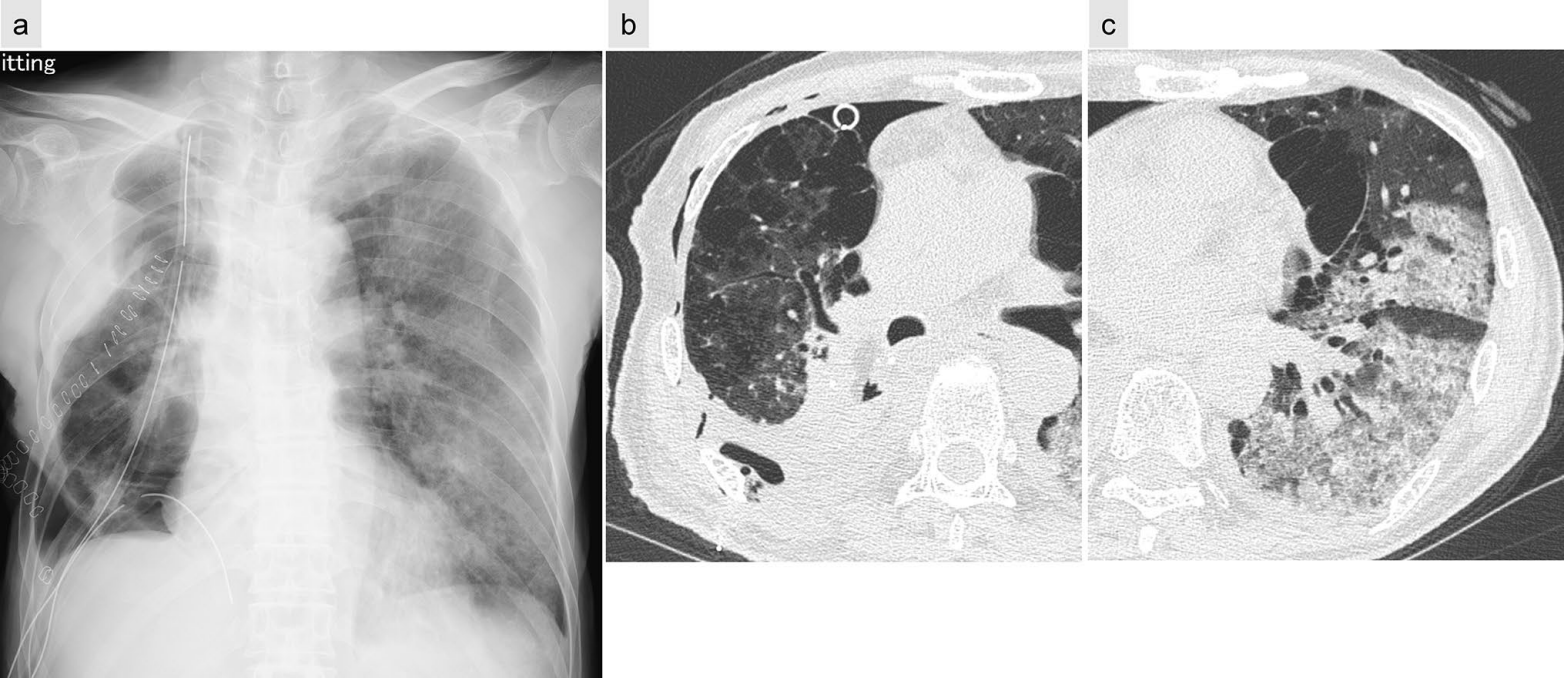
Acute respiratory distress syndrome (ARDS). Twenty-two days following right middle and lower lobe resection, follow-up CXR reveals diffuse ground-glass opacities in the left lung (a). CT imaging demonstrates extensive ground-glass opacities predominantly in the contralateral left lung rather than the postoperative right lung (b, c). Two days earlier, new opacities were noted in the left lung on CXR and CT (images not shown), prompting the initiation of antibiotic therapy. Despite oxygen administration at 8 L/min via face mask, the patient’s hypoxemia persisted, and the left lung opacities progressed. Given the clinical and imaging findings, a diagnosis of ARDS was established
